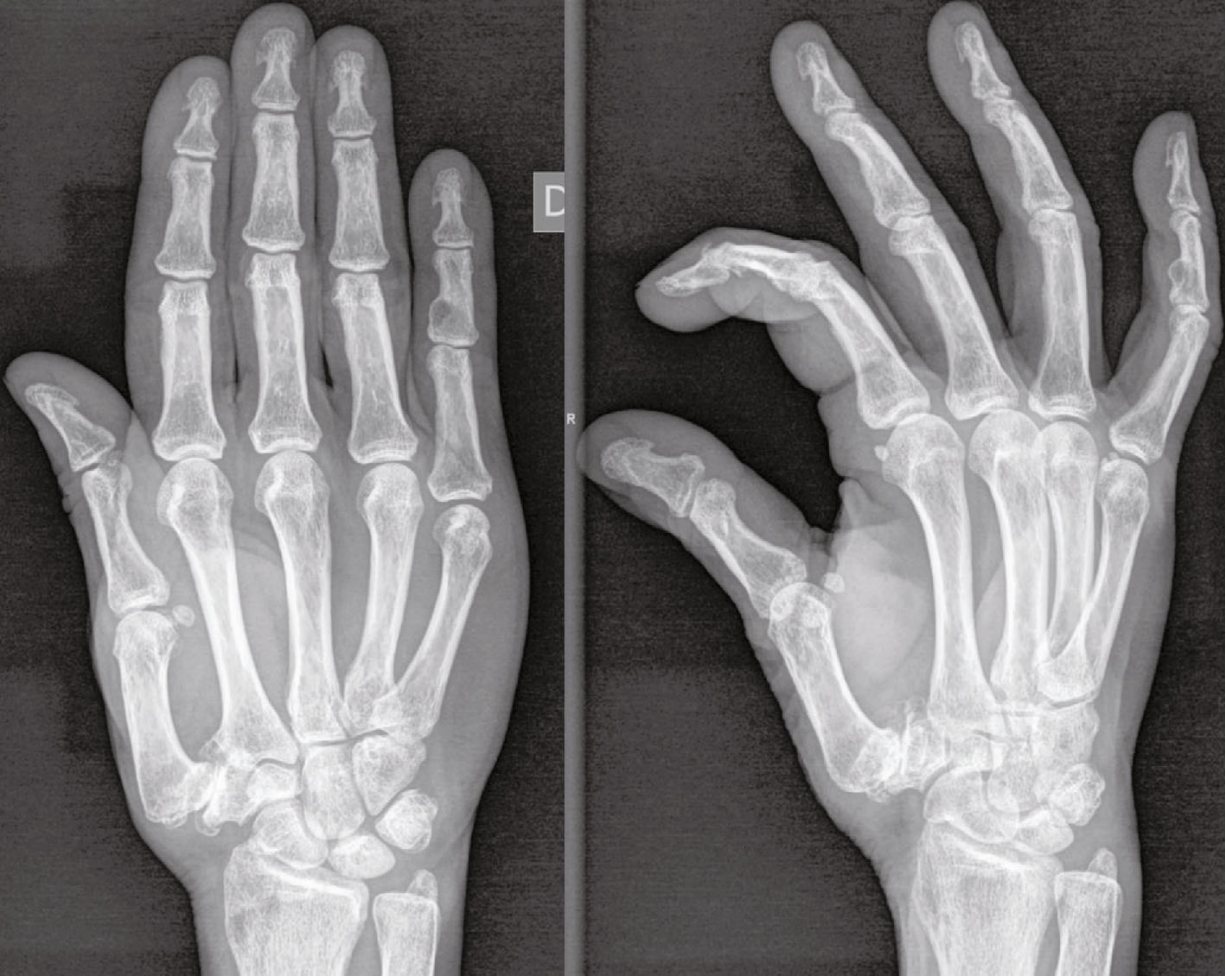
Initial X-ray showing trapeziometacarpal osteoarthritis.
